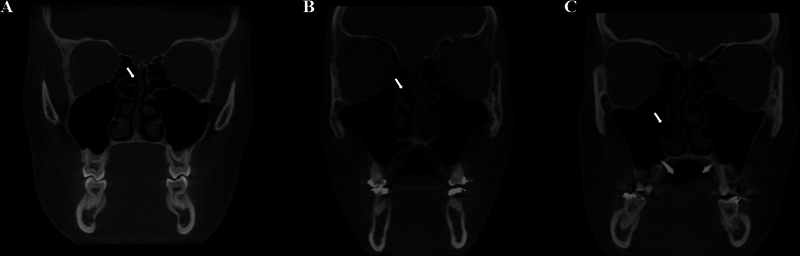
Coronal reconstructions of CBCT exams showing concha bullosa in the three turbinates. (
